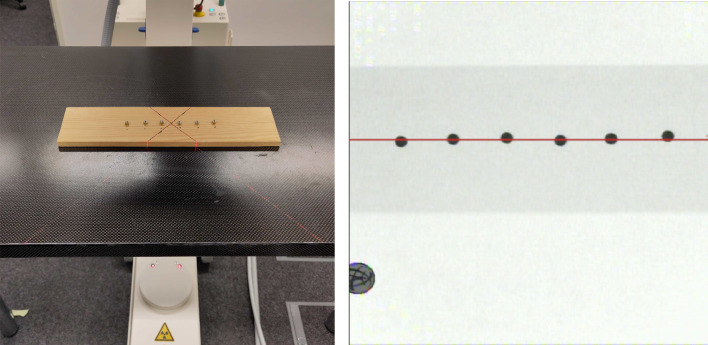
Custom C-arm calibration board under camera (left) and X-ray (right), at a gantry height of $$10\,{\text {cm}}$$ and an angle of $$75^\circ $$. Red line indicates the average center of the metallic orbs, which is used to determine the pivot point of the gantry
